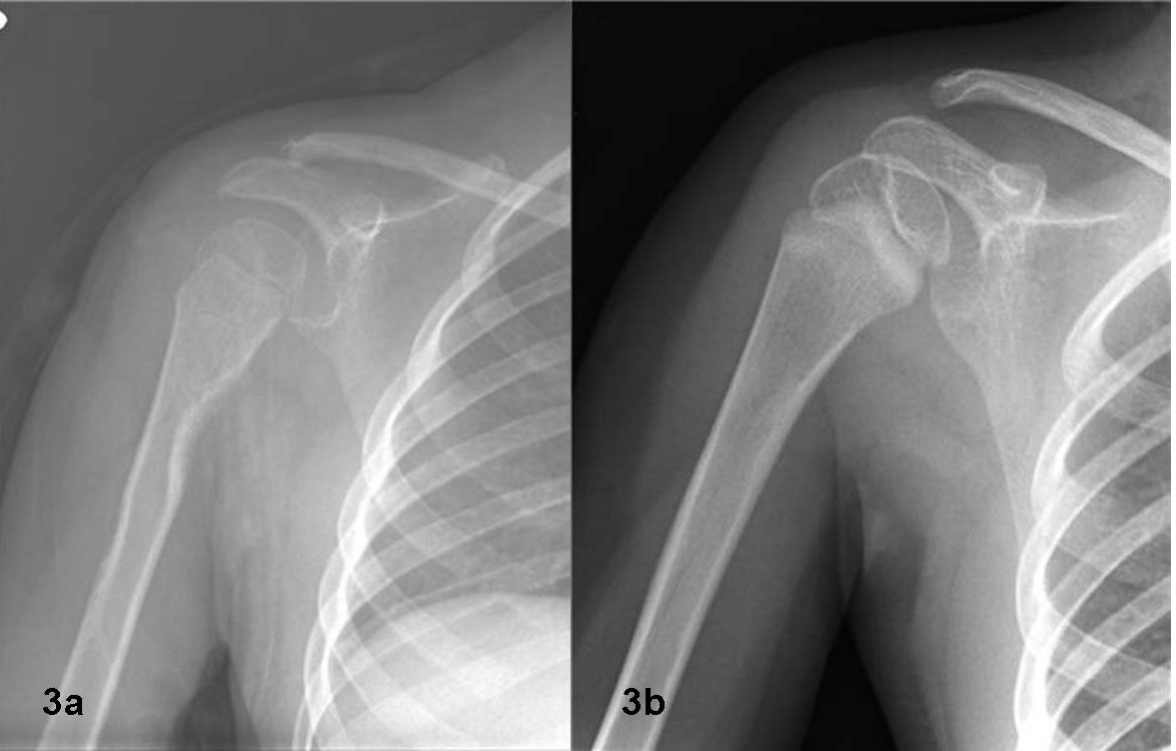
Figures  3a and 3b. X-ray images of the right shoulder show subtendinous resorption of bone of proximal humerus (figure 3A) followed by healing of this lesion (figure 3B) after one year of immunosuppressive medications.
